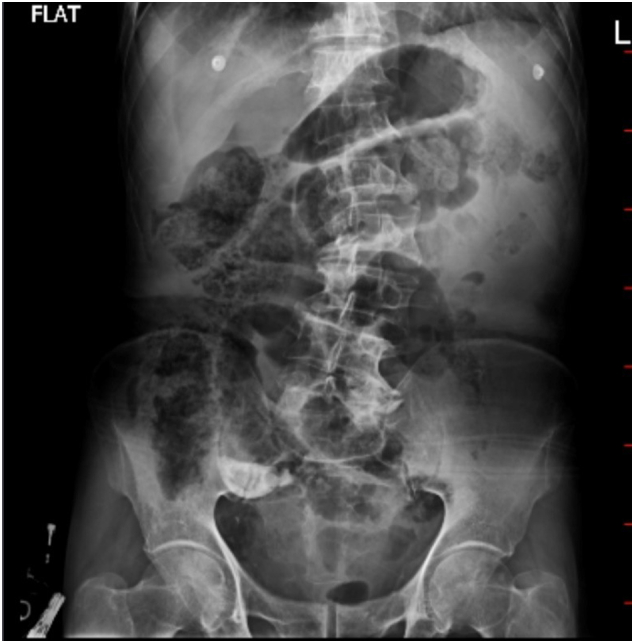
Abdominal X-ray; calcifications in the right lower quadrant.
